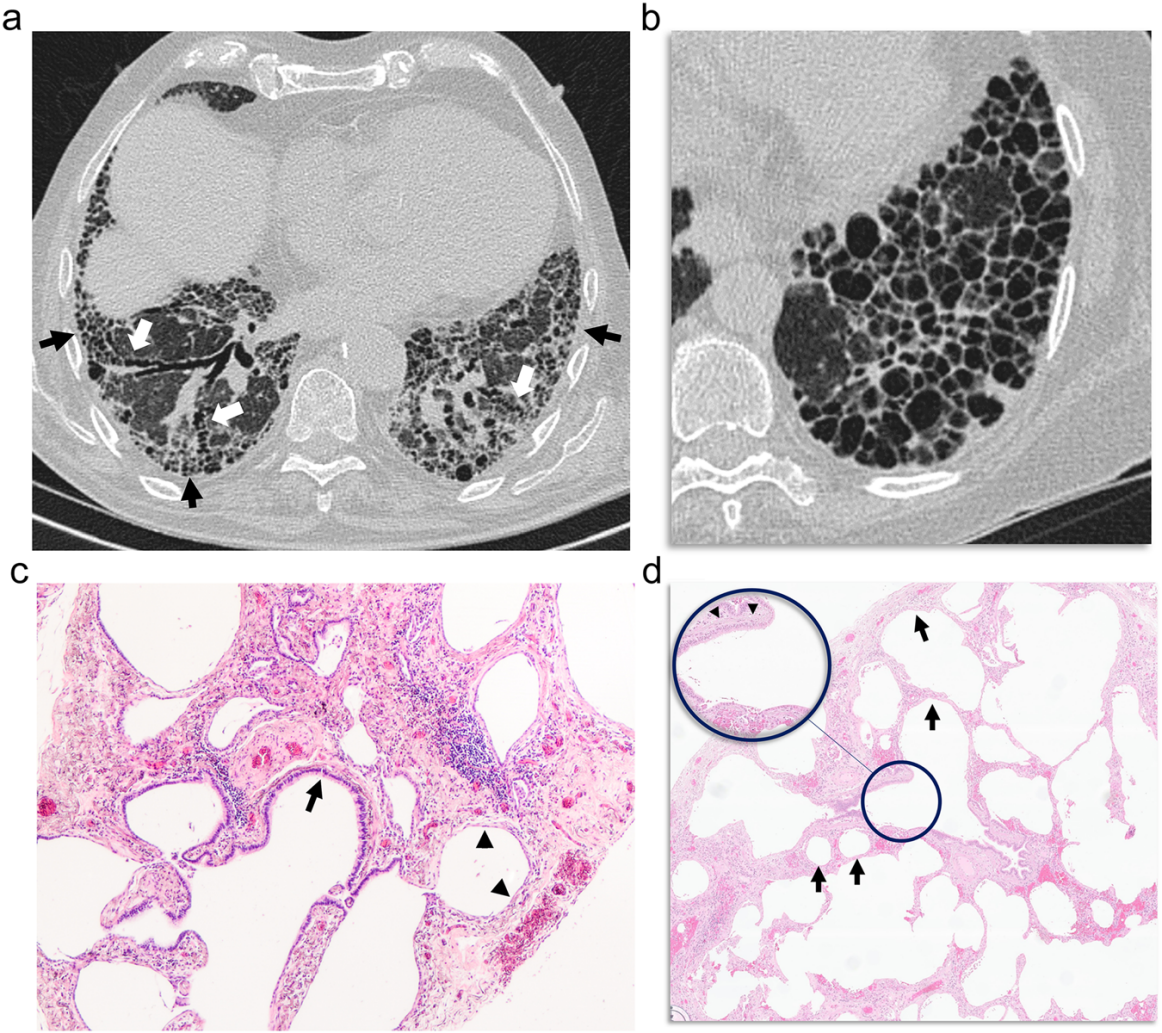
Honeycombing. a CT shows basal fibrosis with small honeycombing cysts (black arrows) consisting of rounded cystic lesions clustered in the subpleural region associated with traction bronchiectasis (white arrows). b Bigger, thick-walled honeycombing cysts sharing their walls are seen. c Pathologically, in samples from other patients, honeycombing is characterized by enlarged airspaces surrounded by fibrosis and lined by bronchiolar (arrow) or hyperplastic alveolar epithelium (arrowheads). d The transition from bronchiolectasis, with bronchial epithelium and a uniform muscular layer (arrowheads in the inset magnification), to respiratory-lined cysts (arrows) is shown
